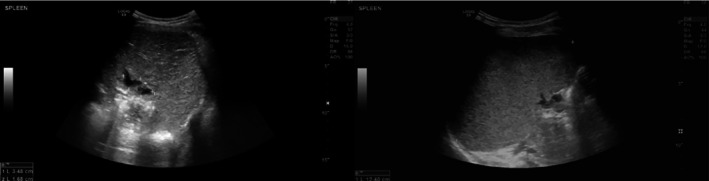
Gray scale ultrasound of the spleen status post operative showing two small located collections in at the site of the operation located at the splenic hilum (a). Follow up imaging showed interval resolution of the previously seen collections with persistent enlargement of the spleen.
